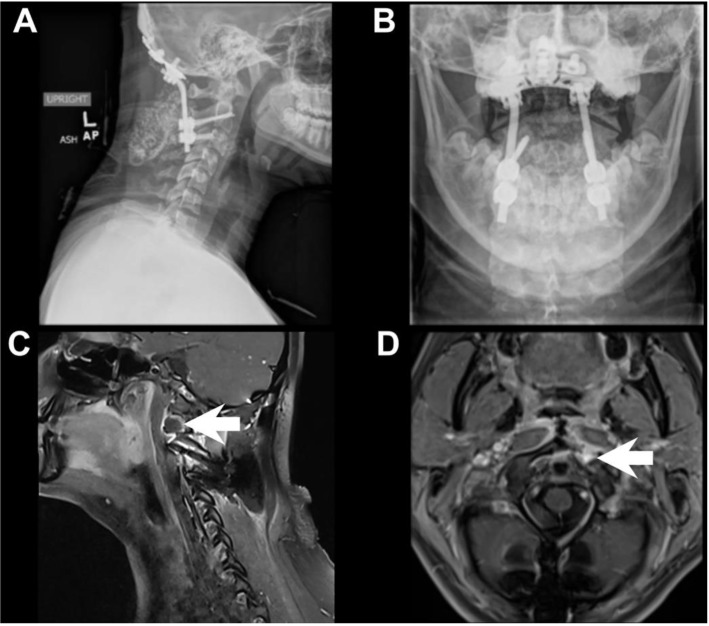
Post-Operative Imaging Studies. Lateral view X-ray of the cervical spine demonstrating hardware on the occiput connected by rods to pedicle screws in C2 and lateral mass screws in C3 bilaterally (A). Open mouth odontoid view X-ray demonstrates normal symmetry of C1 and C2 articulation (B). Post-contrast MRI T1, sagittal view, demonstrates minimal rim-enhancement within the left lateral C1 representing mild residual tumor (white arrow) (C). Post-contrast MRI T1, axial view, of the residual lesion (white arrow) (D).
